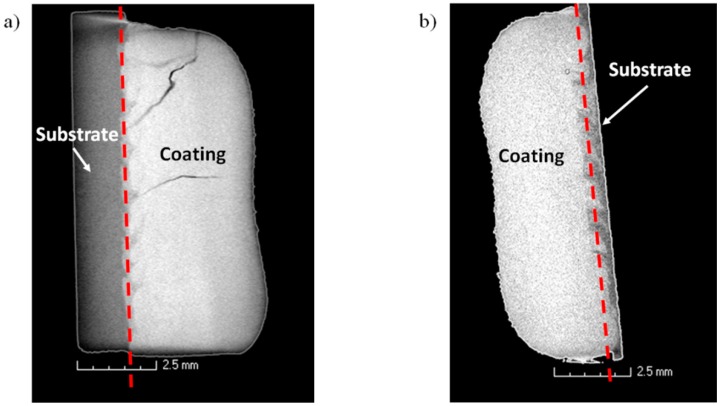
Microfocus X-ray computer tomography image of the LENS-fabricated samples: (a) nr 2 and (b) nr 18.
